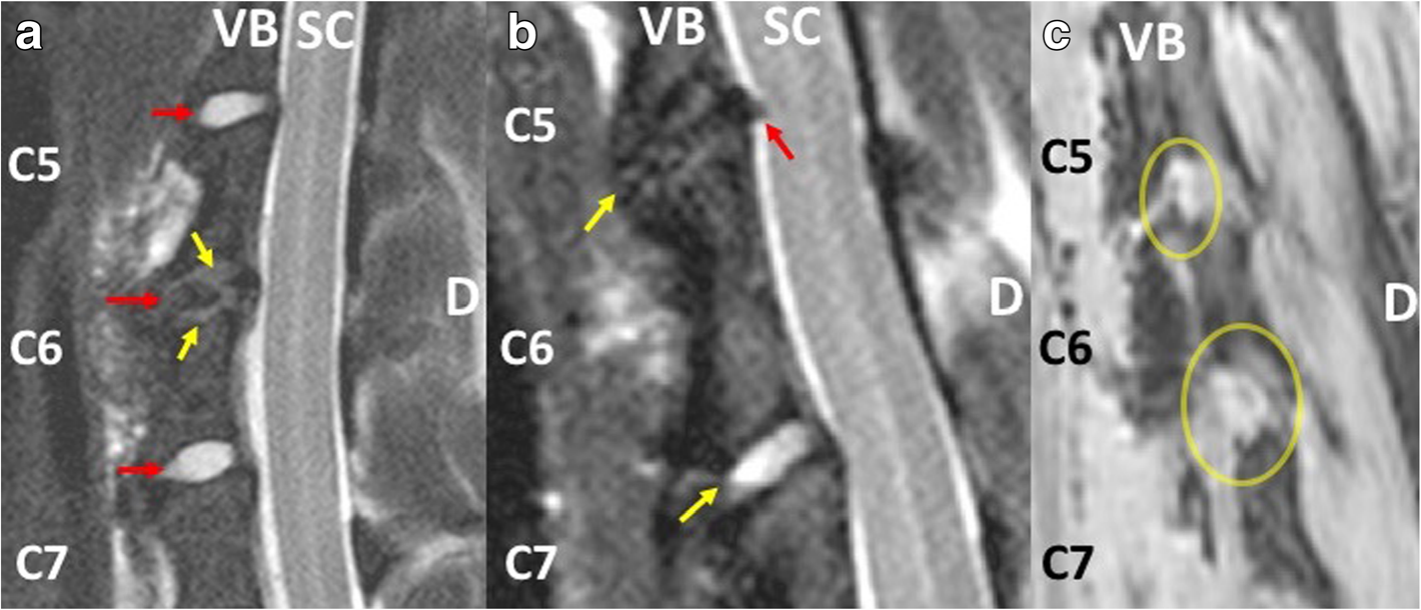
Sagittal T2 weighted MR images of the lower cervical spine. Right side of each slide is dorsal (D) a
